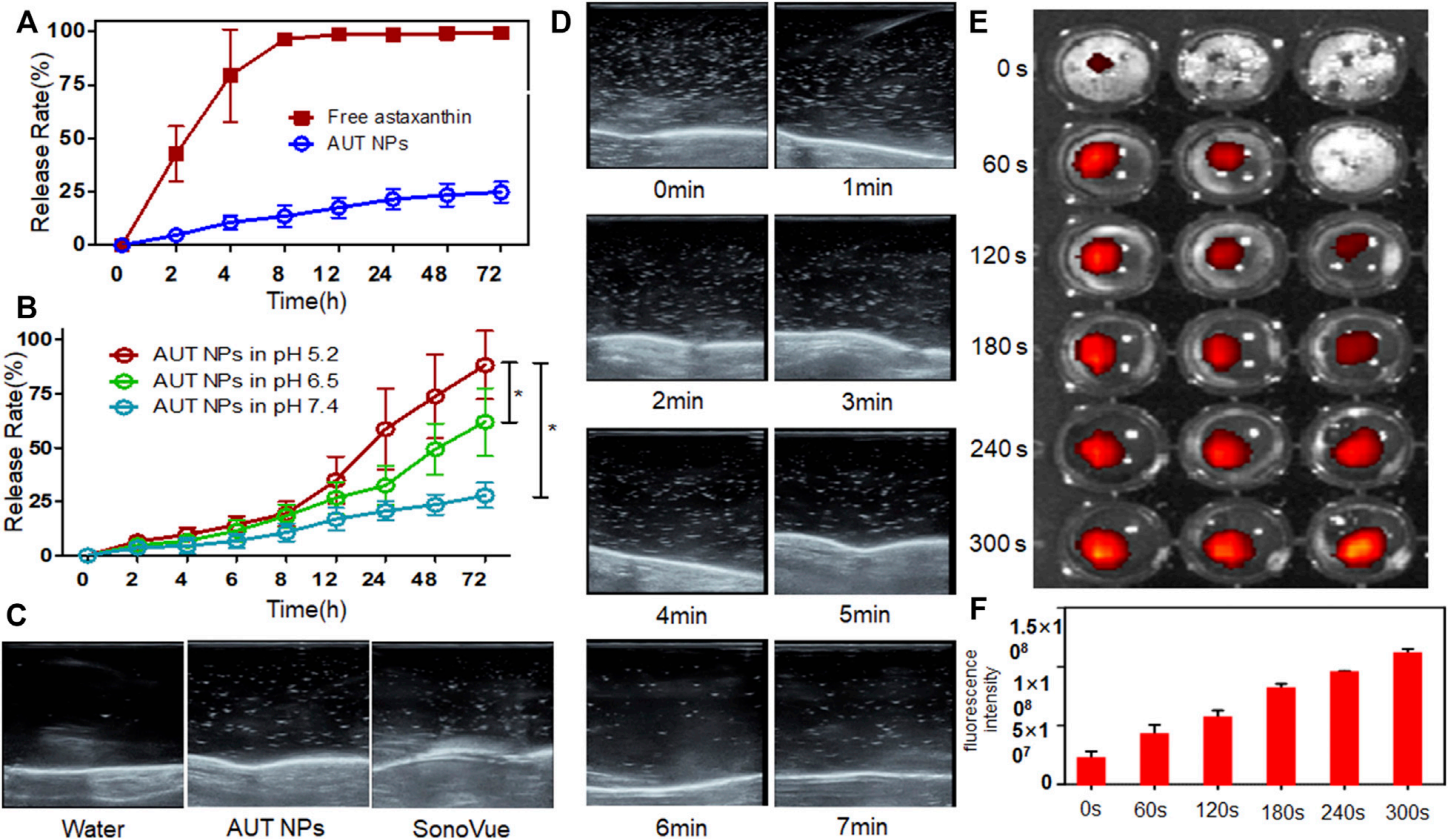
The drug release of AUT NPs under normal (A) and pH stimulus (B). The US triggering images with different contrast agents (C). The real-time ultrasonic images of AUT NPs at each time points (D). The IR780 fluorescent images under US (E). The fluorescent intensity changes of IR780 under a series of US triggering time lengths (F). The* represents *p* < 0.05, (*n* = 3).
